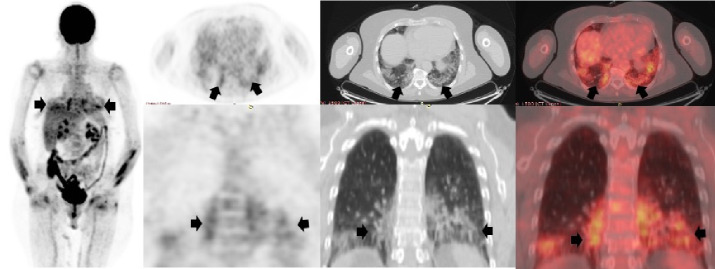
PET/CT con F18-FDG en la que no se observaron lesiones hipermetabólicas relacionadas con la enfermedad de base. Sin embargo, en el tórax se evidenciaron múltiples opacidades en parches con patrón de vidrio esmerilado y actividad metabólica difusa en el lóbulo medio, la língula y en ambos lóbulos inferiores, con SUVmáx de 3,6 (flechas negras), y de aspecto inflamatorio e infeccioso sospechosos de COVID-19.
